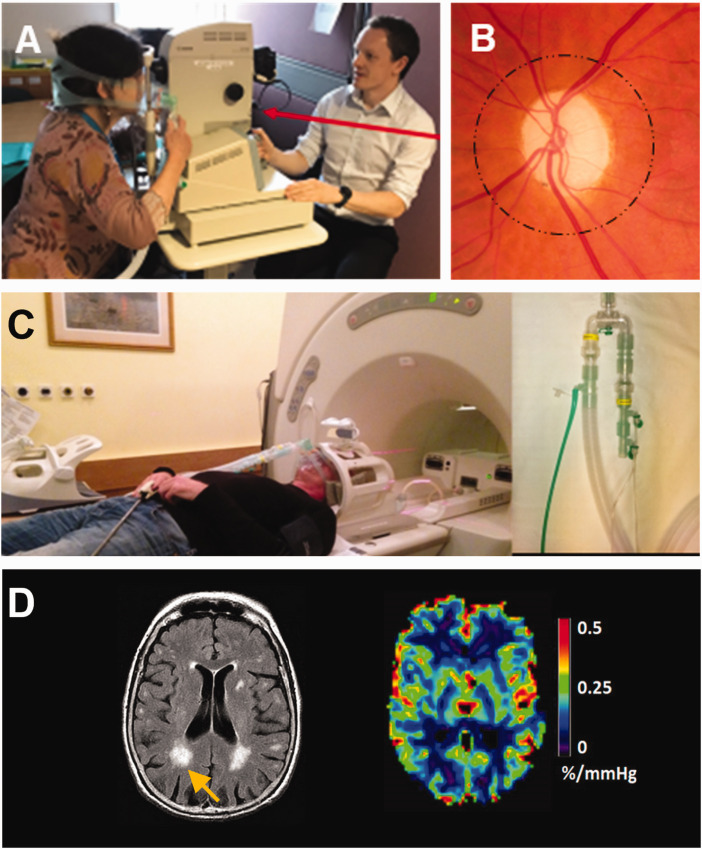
a. Retinal photography performed with a mask and breathing circuit. b. This captures retinal vessels around the optic disc, which are used to calculate the central retinal artery, and central retinal vein equivalents (CRAE, CRVE) and artery-vein ratio (AVR). c. MRI with mask and breathing circuit. D. MRI images show white matter hyperintensities (WMH) among other imaging features of cerebral small vessel disease. BOLD MRI with CO_2_ challenge allows cerebrovascular reactivity (CVR) to be estimated.
